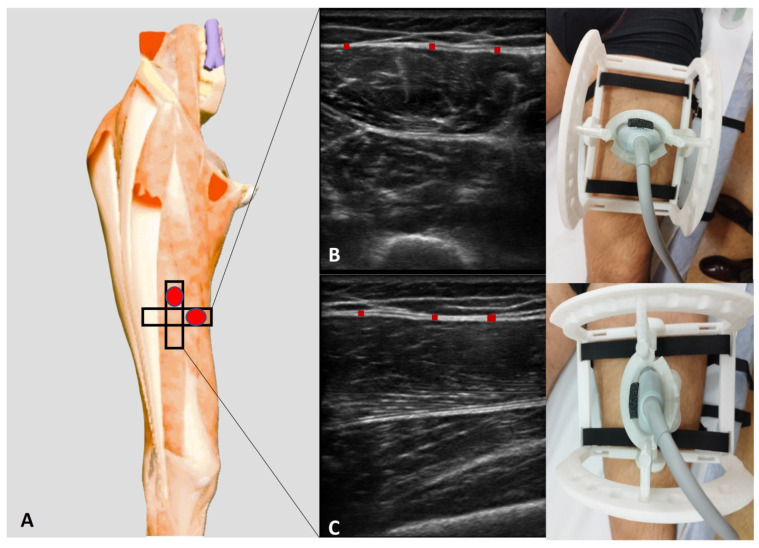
(A) Ultrasound protocol for performing anisotropy assessment of deep fascia. (B) Transversal scan (short axis). (C) Longitudinal scan (long axis). Red rectangle: deep fascia.
